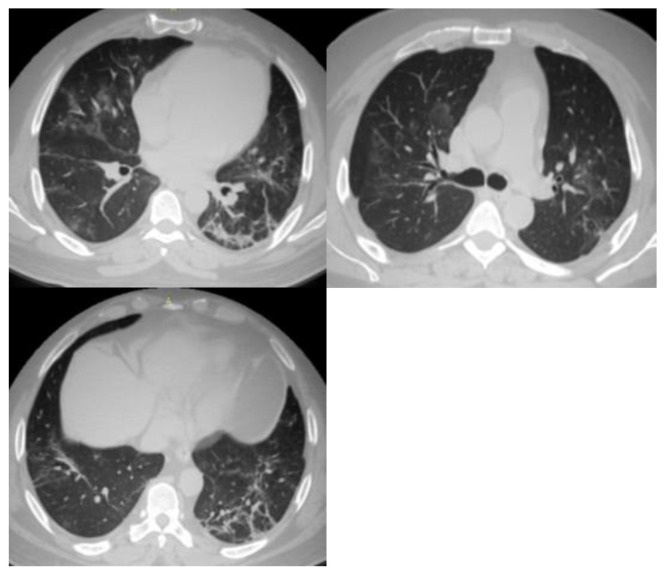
Multiple chest CT images of COVID-19 patients. All observers agreed to calculate the CO-RADS for this case as CO-RADS 5. The agreement between radiologists and pulmonologist was based on the following features: Typical features of multifocal bilateral GGO and consolidation, peri-fissural nodules, perihilar GGO, and centrilobular GGO. In addition to the previous findings, all radiologists agreed on the typical features of multifocal bilateral GGO, consolidation, organizing pneumonia, and thickened vessels.
